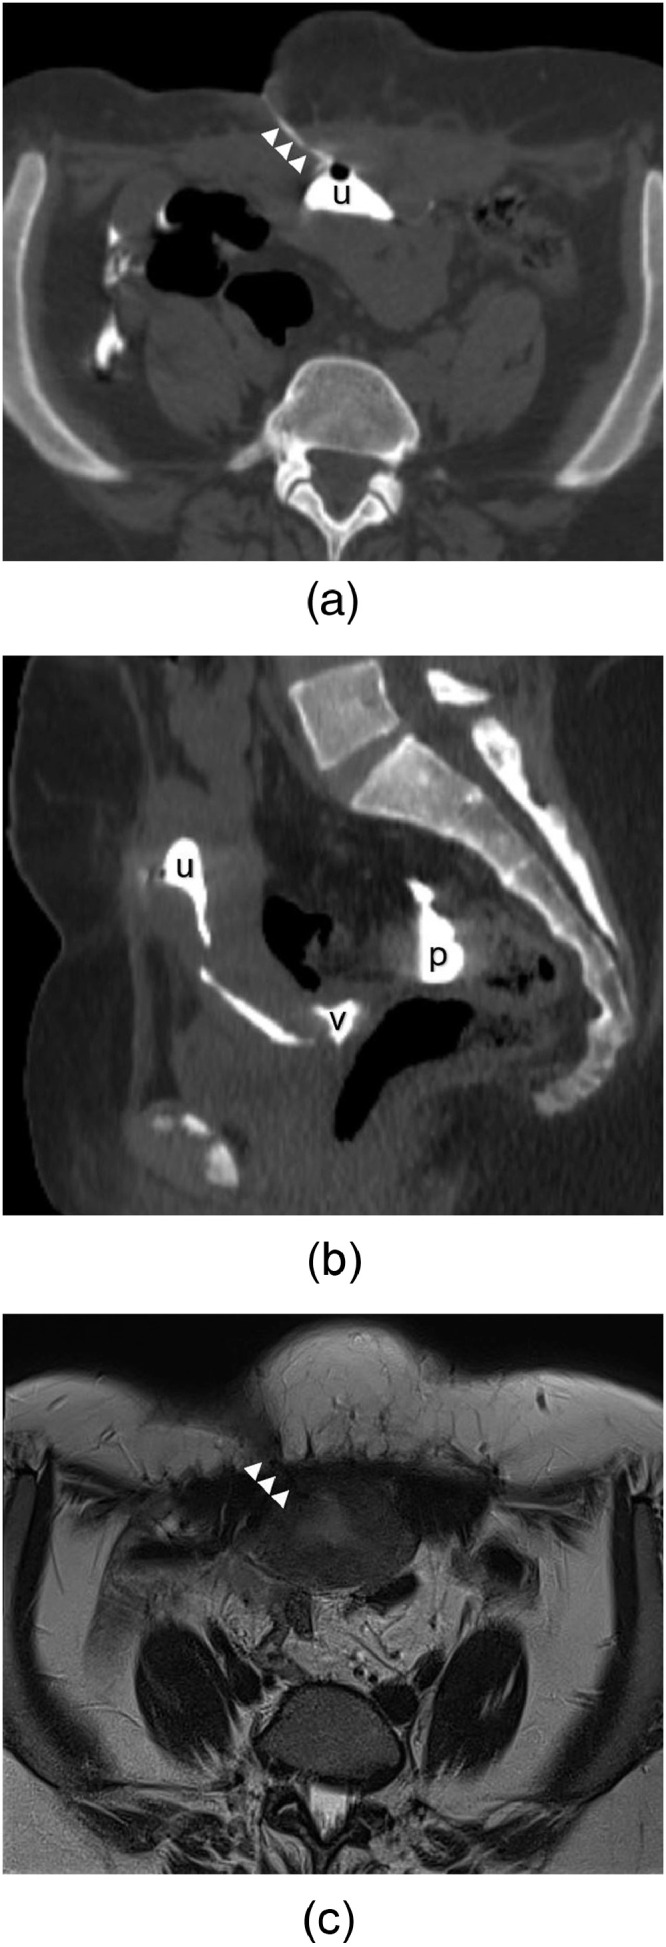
Pelvic CT scan with an injection of diluted iodinated contrast medium through the cutaneous fistulous opening, in the axial (a) and the sagittal (b) planes, showing the opacification of the fistulous tract (arrows) and the uterine cavity (u), with spillage of the contrast medium into the peritoneal cavity through the fallopian tubes (p) and into the vagina through the cervix (v). On pelvic MRI axial T2-weighted sequence (c), the fistulous tract shows a high signal intensity (arrows).
